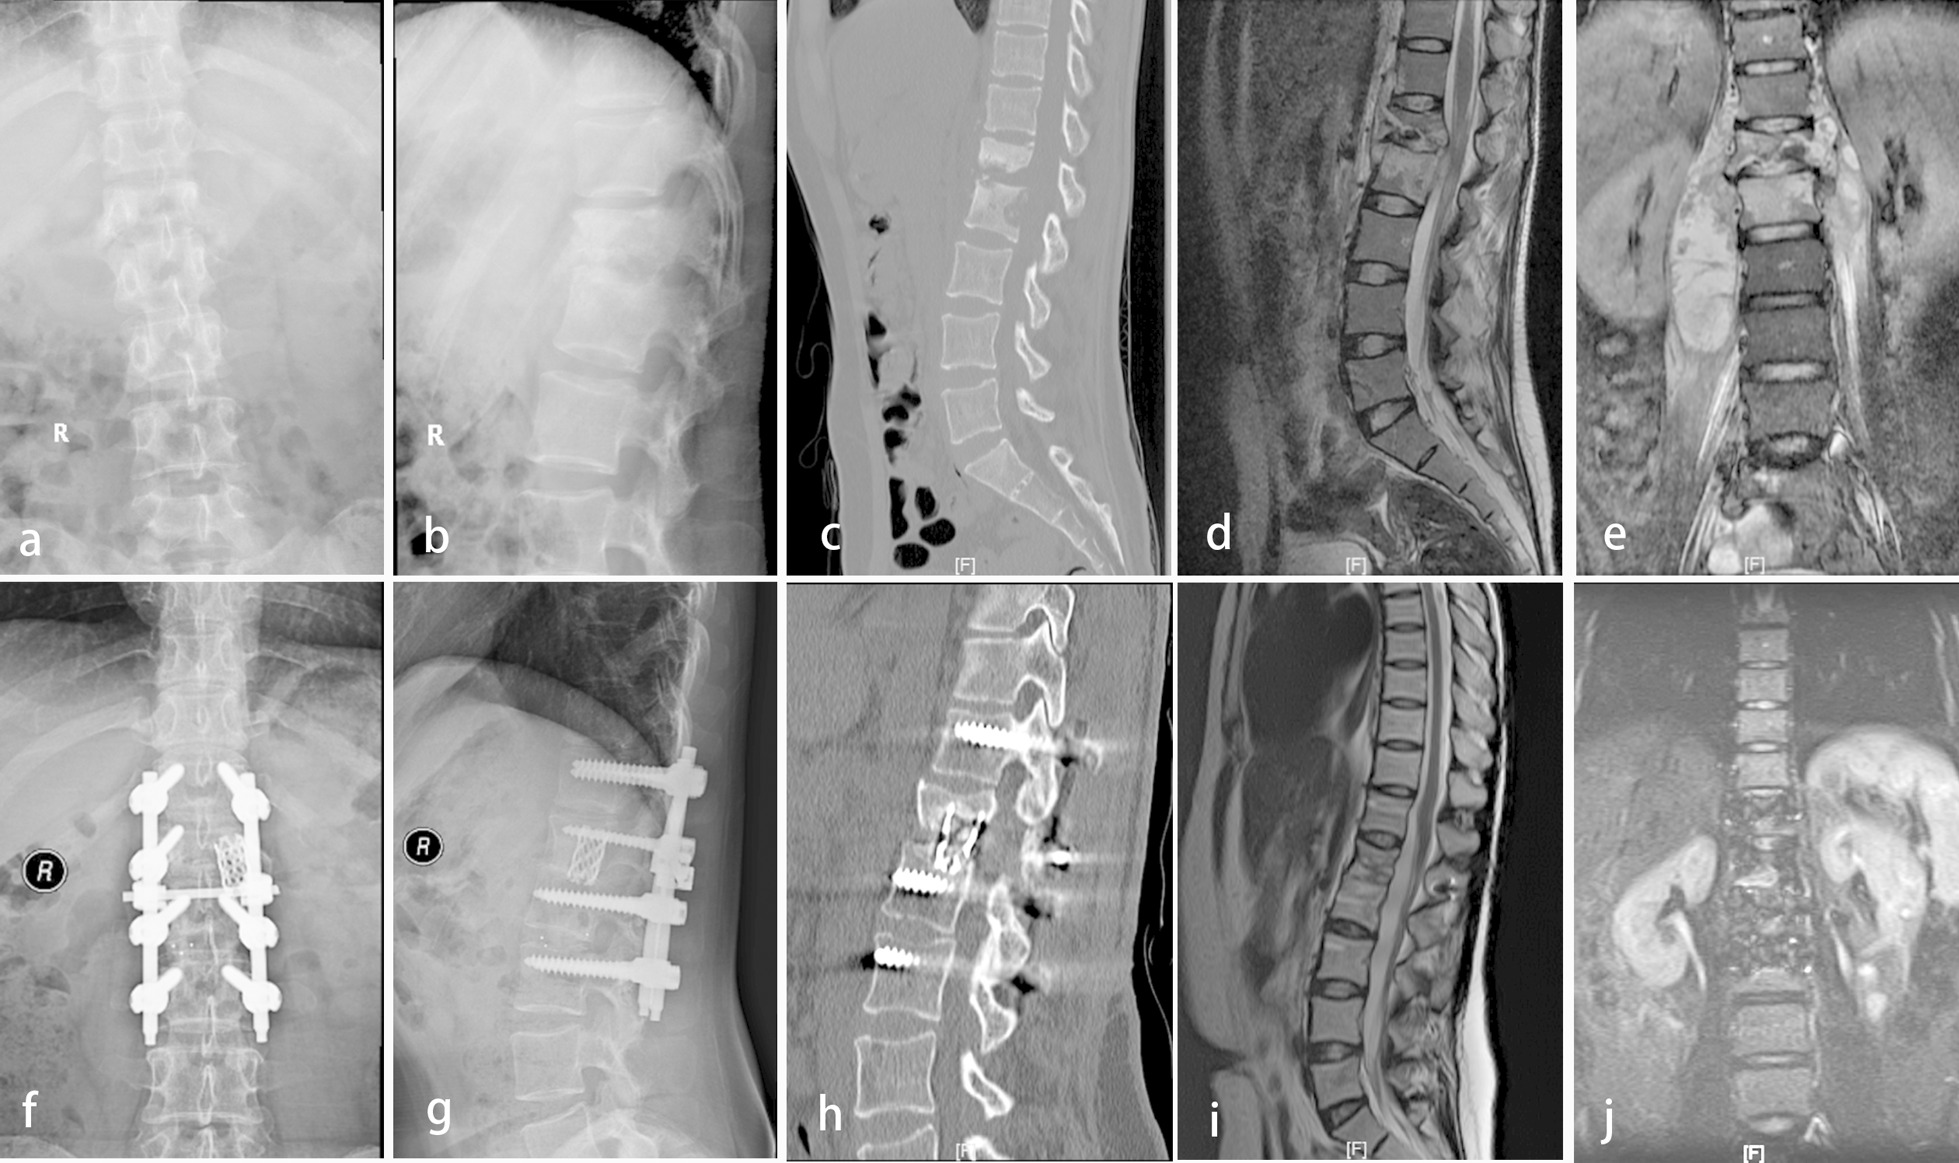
A 27-year-old female patient with thoracolumbar spinal tuberculosis accompany with bilateral psoas abscesses (L1-L2). Preoperative radiography (a and b), CT (c) and MRI (d and e) showed destruction of anterior column and psoas abscesses. Post-operative radiography (f and g) and CT (h) showed bone fusion achieved with no screw loosening or rod fracture appeared at the final follow-up. Post-operative MRI (i and j) showed psoas abscesses disappeared at the final follow-up
